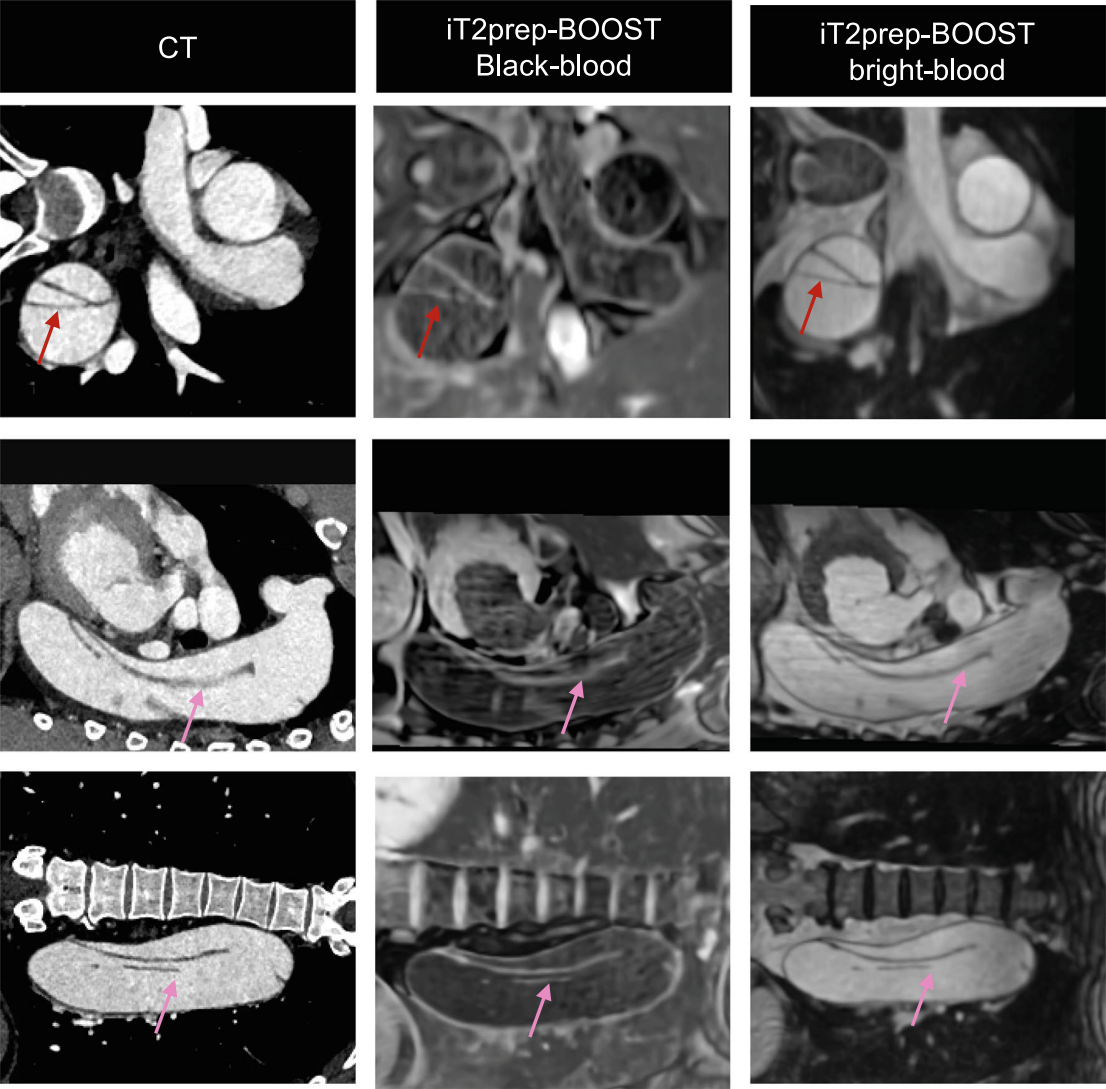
Comparison between conventional CT and proposed iT2Prep‐BOOST MR imaging in 54‐year‐old patient with chronic aortic dissection. The dissection flap is complex and extends from the aneurysmal thoracic descending aorta to the left common iliac artery (pink arrow). It is demonstrated both in the proposed iT2prep‐BOOST and the CT images (red arrow). The false lumen is dilated and aneurysmal.
